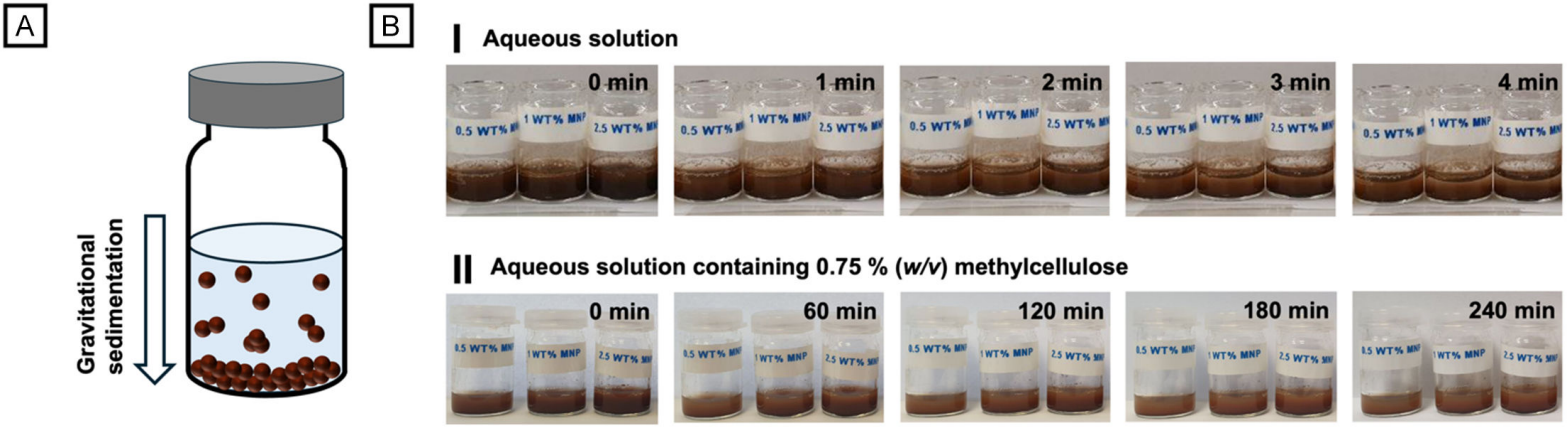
Sedimentation of MNPs over time in aqueous suspensions. (A) Scheme of gravitational sedimentation in aqueous suspension driven by the MNPs’ high density. (B) Comparative screening of sedimentation over time with varying concentrations of MNPs ranging from 0.5% to 2.5% (*w/w*) after 10 min of ultrasonication treatment in I) DI water and II) DI water supplemented with 0.75% (*w/v*) methylcellulose.
